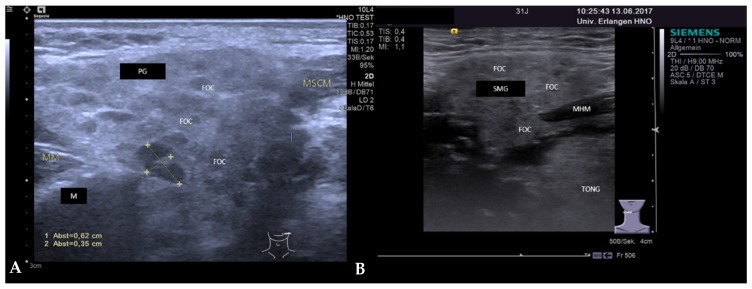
(A,B): Ultrasound findings at the early stage of M. Sjögren in the PG (A) and SMG (B). The parenchyma is slightly hypoechoic with multiple small (size 2–3 mm) and more pronounced hypo-echoic foci, which correspond to an accumulation of lymphatic cells. Glands are moderately enlarged. Abbreviations: PG, parotid gland; SMG, submandibular gland; M, mandible; MM, masseter muscle; MHM, mylohyoid muscle; MSCM, sternocleidomastoid muscle; TONG, tongue; FOC, Focus.
